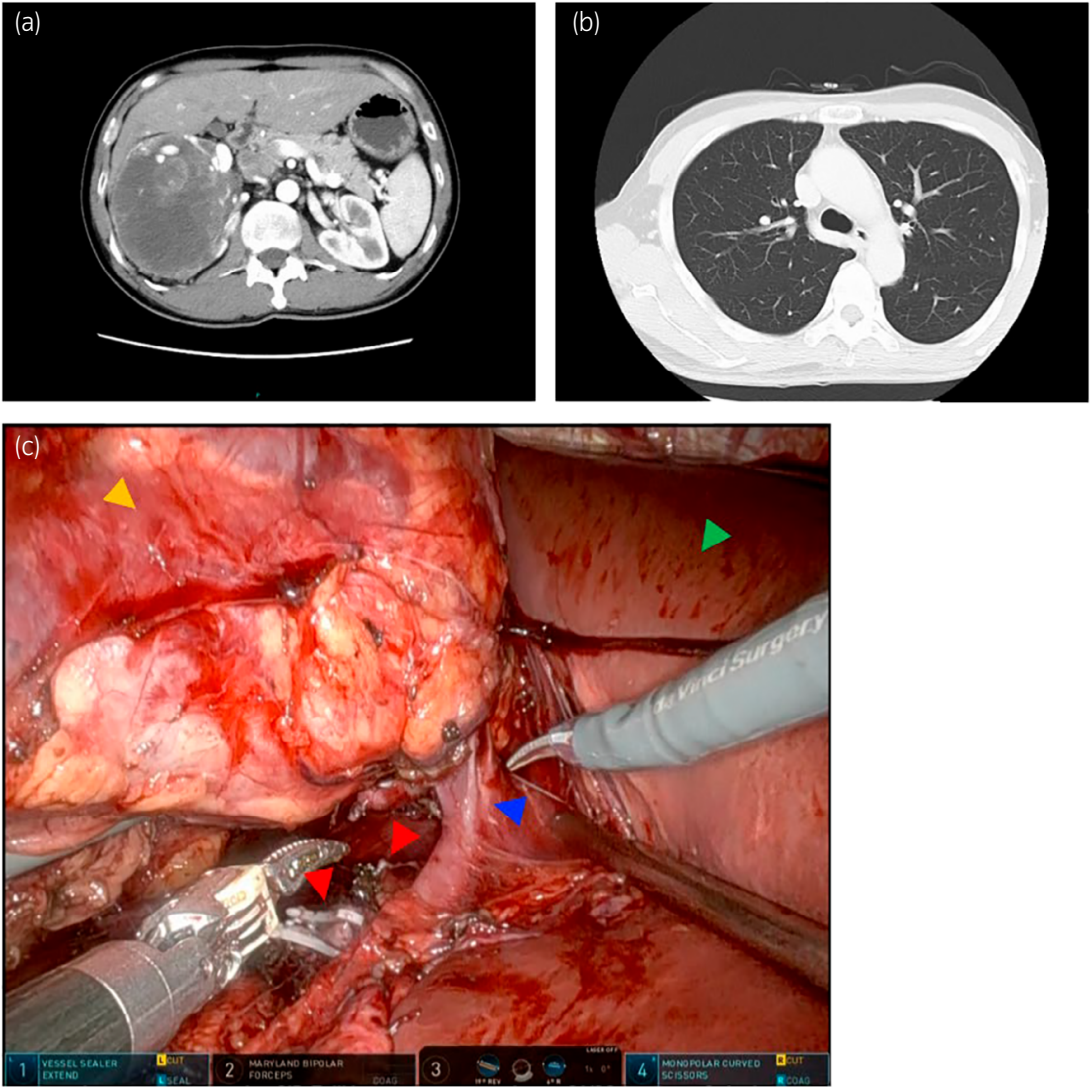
Clinical findings during the systematic treatment. (a) Abdominal enhanced CT performed in 19th week after the initiation of lenvatinib plus pembrolizumab combo. Primary tumor shrunk remarkably into 13 cm in diameter. (b) Disappearance of lung metastases was confirmed by chest CT. (c) Representative image from the surgical video. Primary tumor (yellow arrow), ligated and dismembered renal arteries (red arrows), renal vein (blue arrow), and liver (green arrow) were demonstrated during the robot‐assisted laparoscopic procedure.
